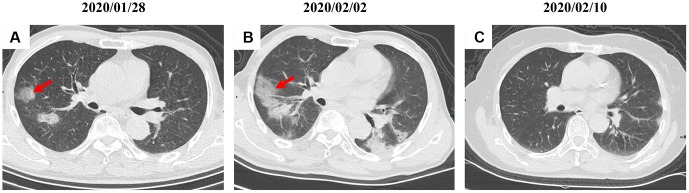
A serial CT images after admission of a 54-year-old male patient. (A) Patch ground-glass opacity was observed in the middle right lobe. (B) 5 days later, significant larger patch ground-glass opacities were observed in bilateral lungs. (C) Follow-up CT scans on day 13 after admission show a remarkable improvement. Typical lesions were marked with red arrows.
